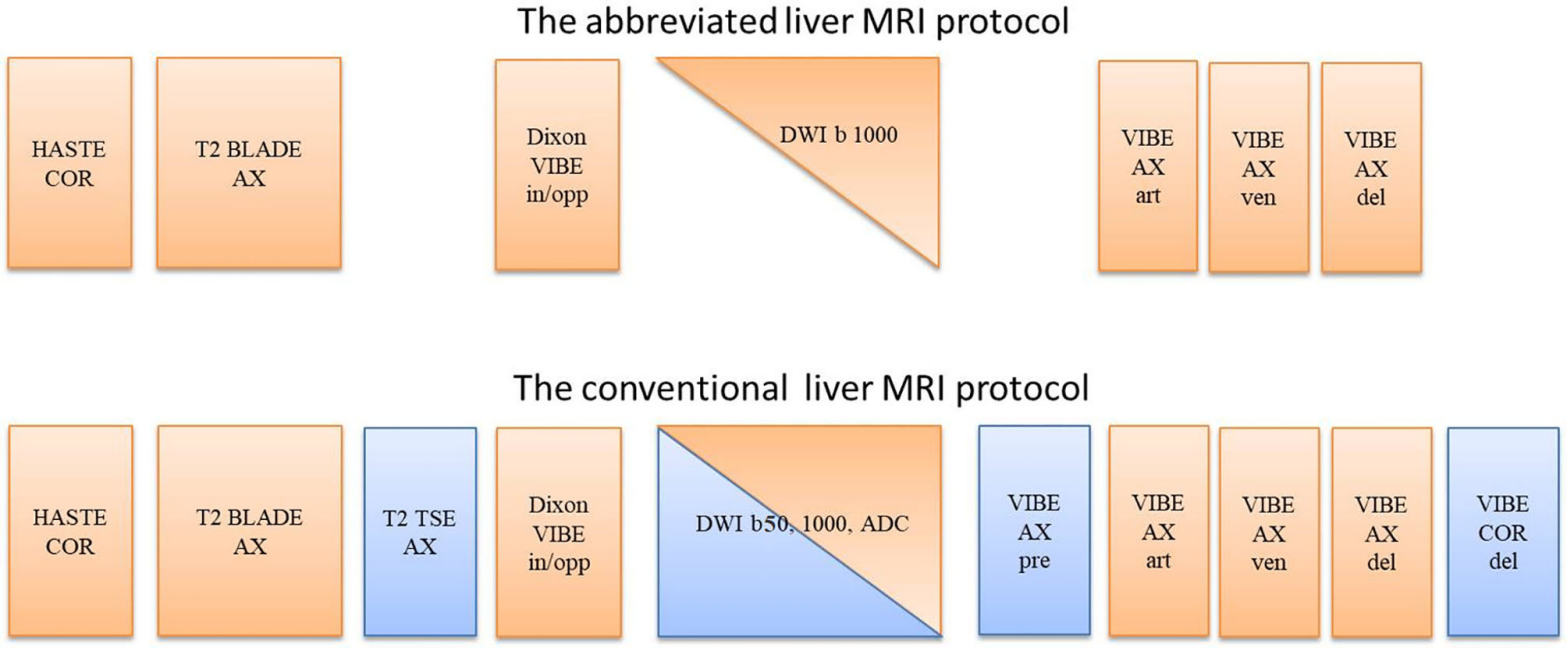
The sequences used in the abbreviated fast liver MRI protocol and the conventional liver MRI protocol. COR, coronal; AX, axial; in/opp, in-phase and opposed-phase; art, arterial phase; ven, venous phase; del, delayed phase.
